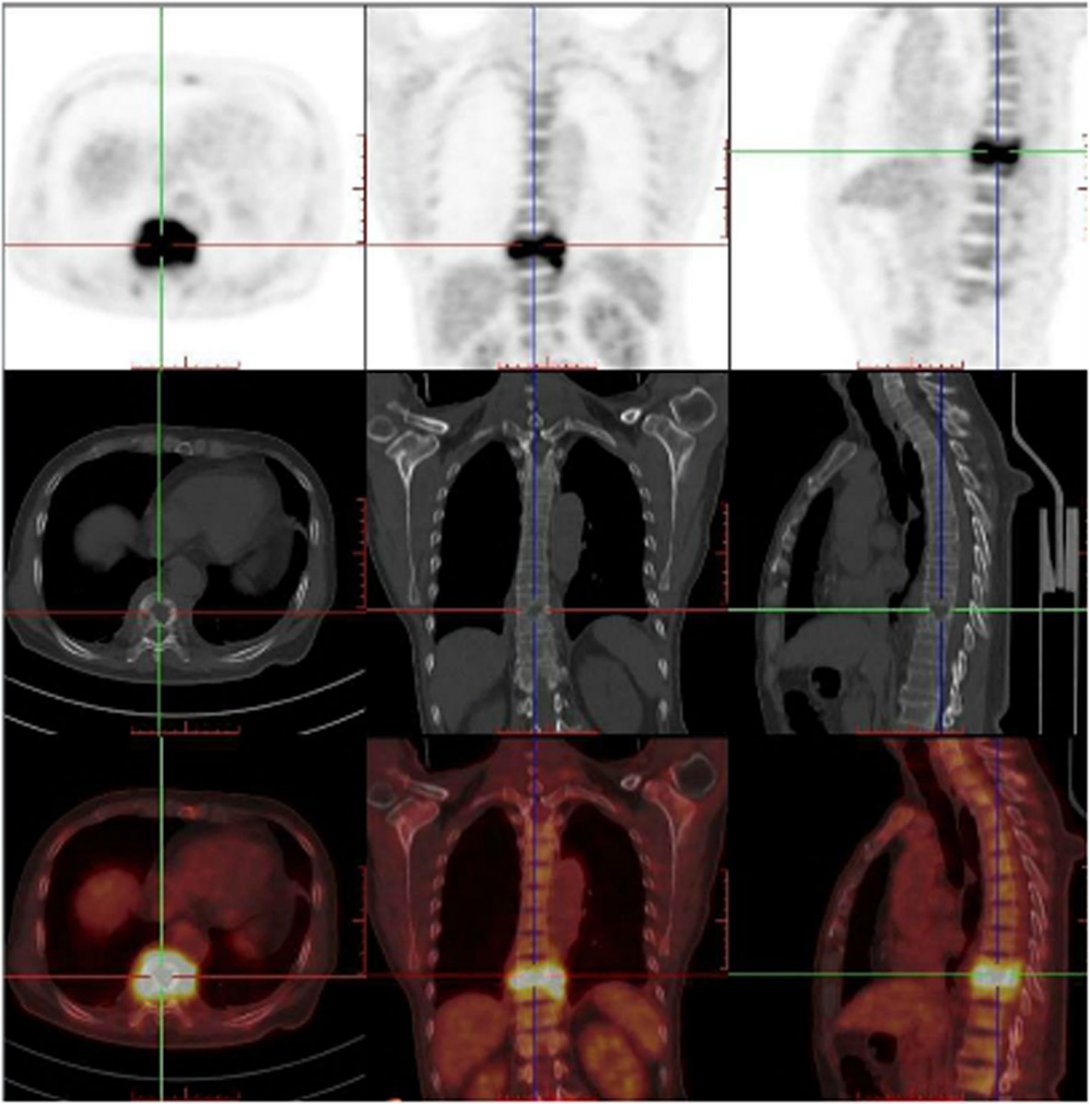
PET-CT images before operation. PET-CT showed changes in T9-T10 vertebrae and intervertebral space, formation of soft tissue masses adjacent to the vertebra, and increased fluorodeoxyglucose (FDG) metabolism
